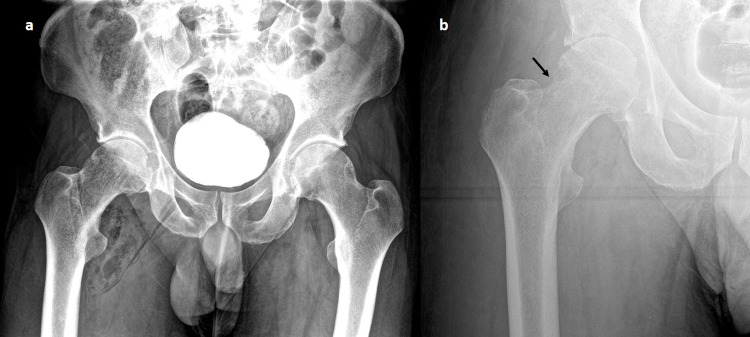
(a) Initial plain film showing gas within the femoral head and the soft tissue. X-ray was acquired shortly after the CT, thus contrast within the urinary bladder. The needles in the groin are very subtle. (b) Plain film eight weeks later shows the resolution of the gas. A subtle cortical defect is seen in the lateral aspect of the femoral neck secondary to the infection (arrow).
